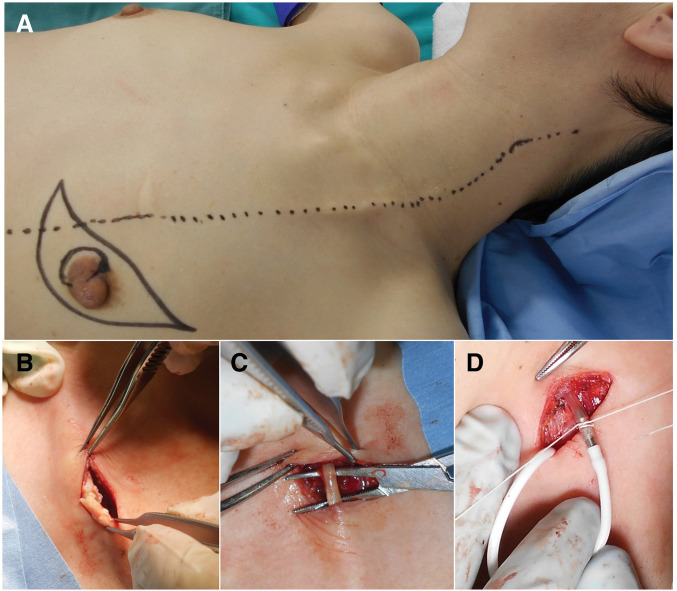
Surgical findings. (A) The thoracic course of the VP shunt was marked on the skin via direct visual inspection and ultrasound guidance prior to surgical draping. (B) The planned connection site above the clavicle was calcified, and a partial rupture occurred during traction. (C) By shifting the skin incision cranially and tracing the catheter upward, an area without calcification, where the catheter’s elasticity was preserved, was identified and chosen as the new connection site. (D) The existing and new catheters were connected using an I-shaped titanium connector.
